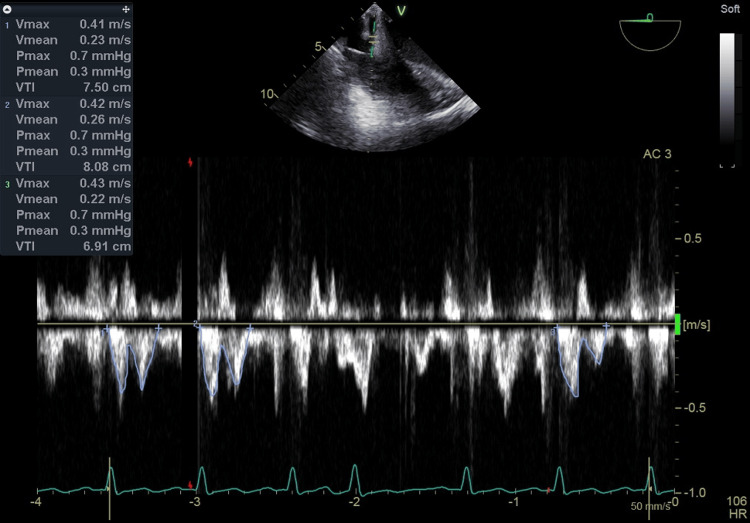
The VTI of CS blood flow measured by the TEE pulse Doppler immediately after weaning from CPB The VTI value after weaning from CPB was 7.50 cm (HR 106 beats/min) calculated as the average of three heartbeats excluding premature contractions. In addition, the TEE setting was set to Frames per second 9, Frequency 3.1 MHz, Power 0 dB, Gain -2 dB, Velocity scale 2.0 m/s, Sample volume 4.0 mm, Sample volume depth 2.7 cm and Sweep speed 50 mm/s. VTI: velocity time integral, CS: coronary sinus, TEE: transesophageal echocardiography, CPB: cardiopulmonary bypass
